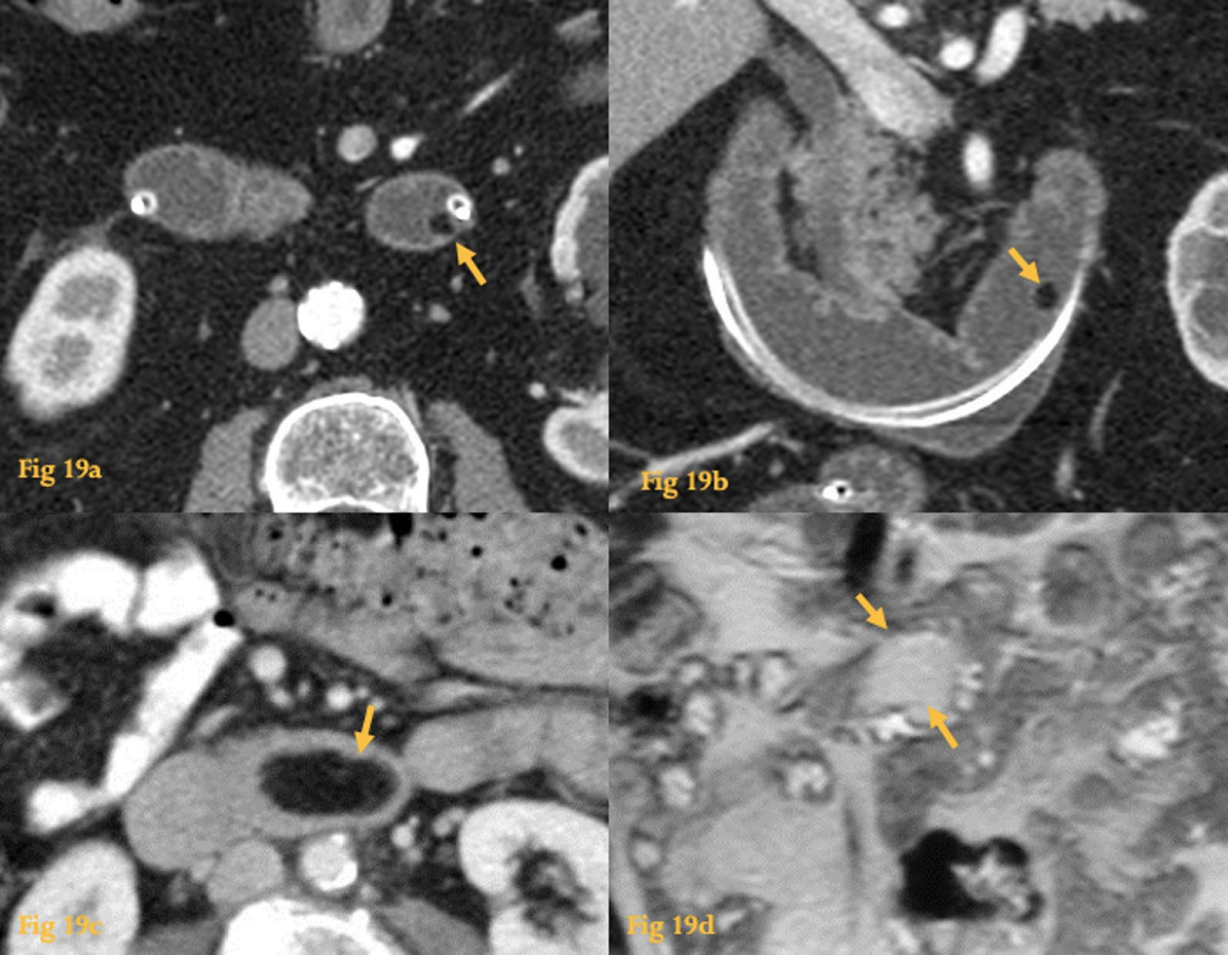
Lipoma. CT enteroclysis, axial (a) and coronal reformation (b) a small endoluminal nodule, with fat density is seen protruding of the wall at D4 (arrows). Abdominal CT of another patient (c) shows a duodenal mass with homogeneous fat attenuation in the horizontal duodenum (arrow). MR coronal HASTE image (d) shows the mass has high signal intensity (arrows), equal to the mesenteric fat
